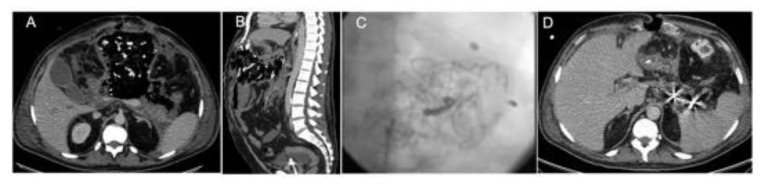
Splenic artery trans-arterial embolization by the placement of coils in a patient with acute pancreatitis patient submitted to an “open abdomen” tamponade for hemorrhage control. (A) Axial CT view, (B) sagittal CT view, (C) angiographic view post-embolization, and (D) axial CT control after embolization, with metal artifacts from the coils placed in the splenic artery.
